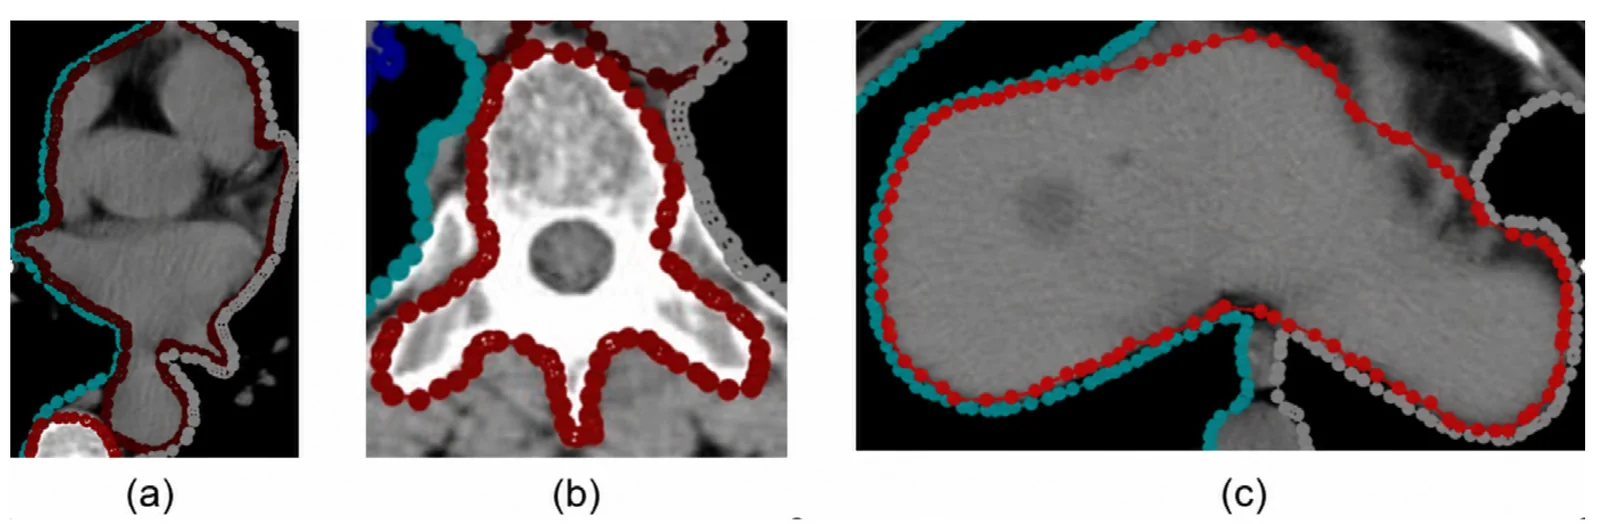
Examples of dynamically labeled anatomical structures in computed tomography (CT) images used as context for the object detection model. (a) shows different morphological representations of the heart. (b) presents an axial slice with the spinal cord structure (Spinal_cord class) delineated. (c) presents an axial slice highlighting a structure categorized as part of the digestive region. The different colors are used only for representative visualization of the manually delineated anatomical structures.
